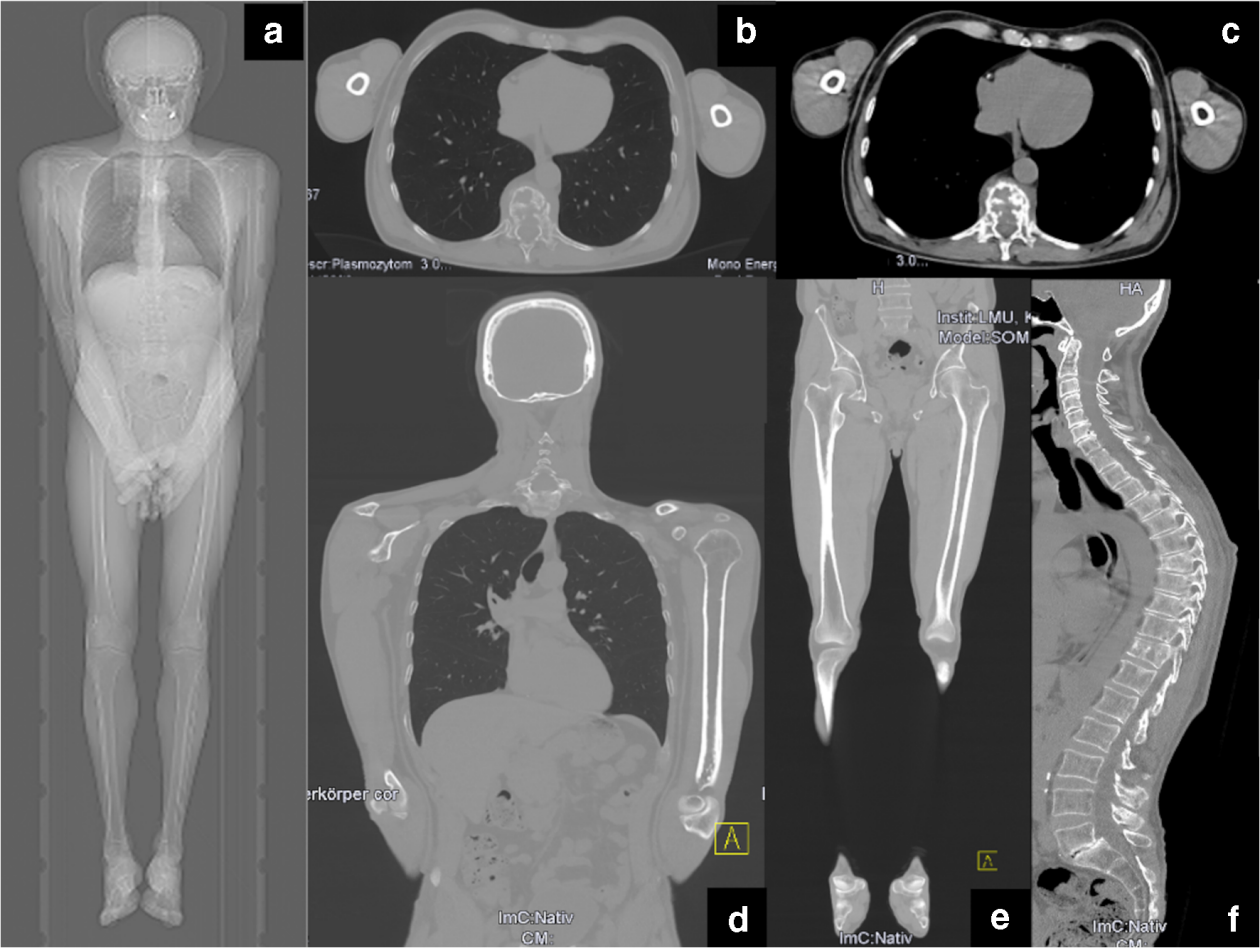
Image examples of a whole-body low-dose CT scan (a: scout). Images were reconstructed in axial 3 mm slices using the intermediate (b) and soft-tissue kernel (c) in order to evaluate soft-tissue masses and the intrathoracic and intra-abdominal organs. The skeleton is further reconstructed in coronal 3 mm slices using the bone kernel for the reading of the upper (skull, thorax, arms; b, *d*) and the lower parts (pelvis, legs; e) of the body. The spine is additionally reconstructed in sagittal orientation (f).
